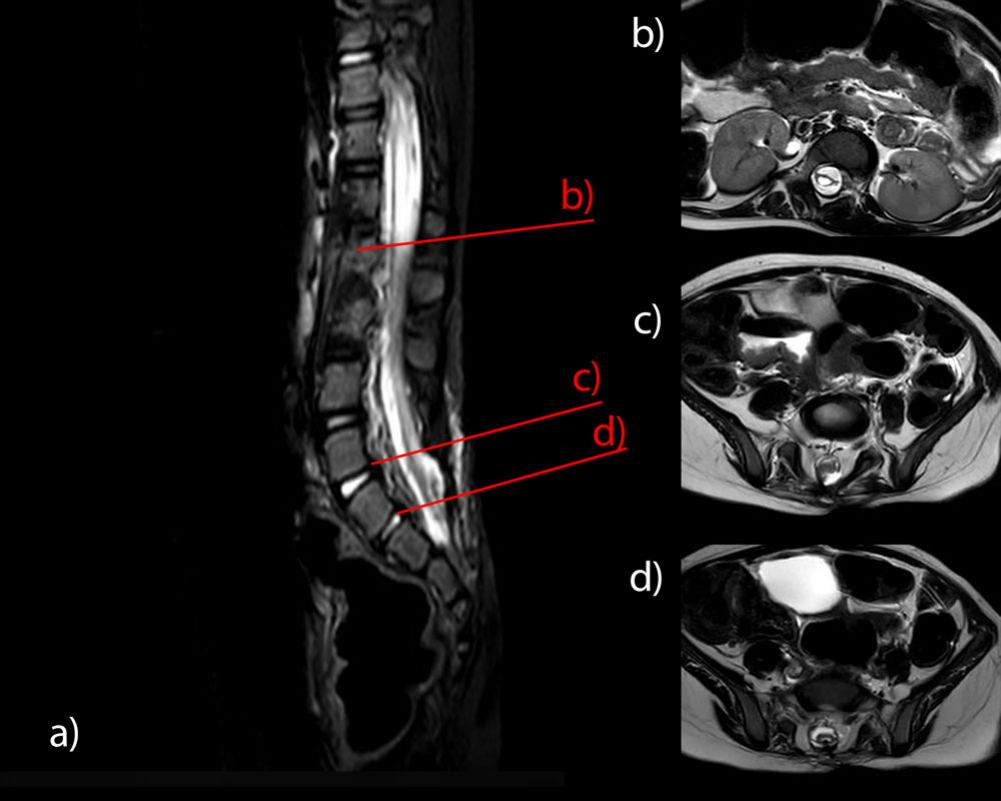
Post‐operative sagittal T2 (a) and corresponding Axial T2 MRI slices (b–d) at day 5: At level of T11 with reduced syringomyelia, L5 with removed lipoma and S1/2 with placode neurulation after dermoid removal with residual air inclusion.
